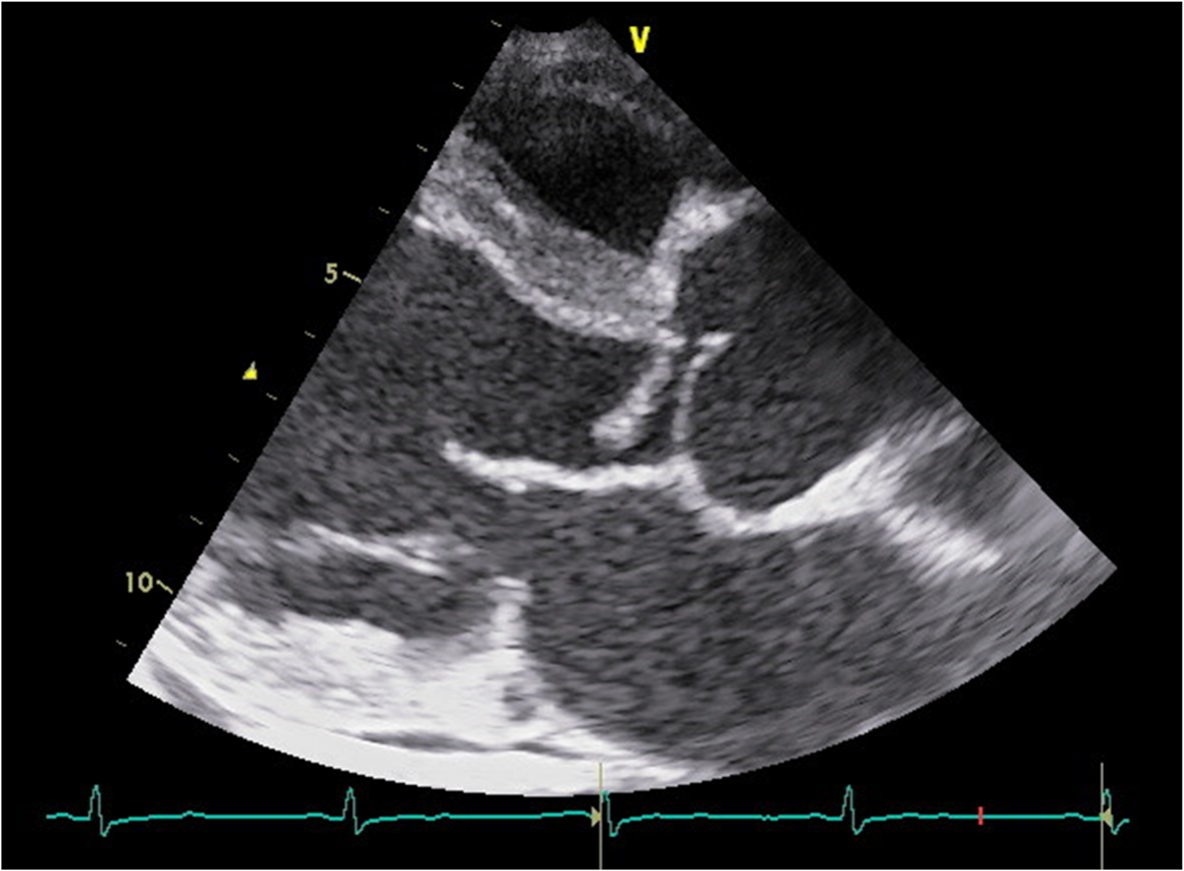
Echocardiogram showing moderate-to-severe aortic valve regurgitation and vegetation of 17-mm in size
